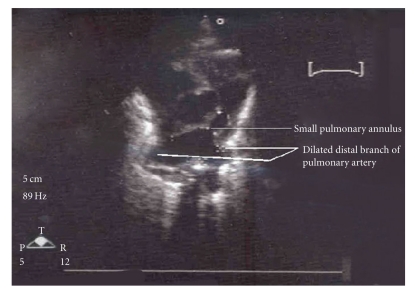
Echocardiogram of Twin A showing small pulmonary annulus and dilated distal branch of pulmonary artery.
